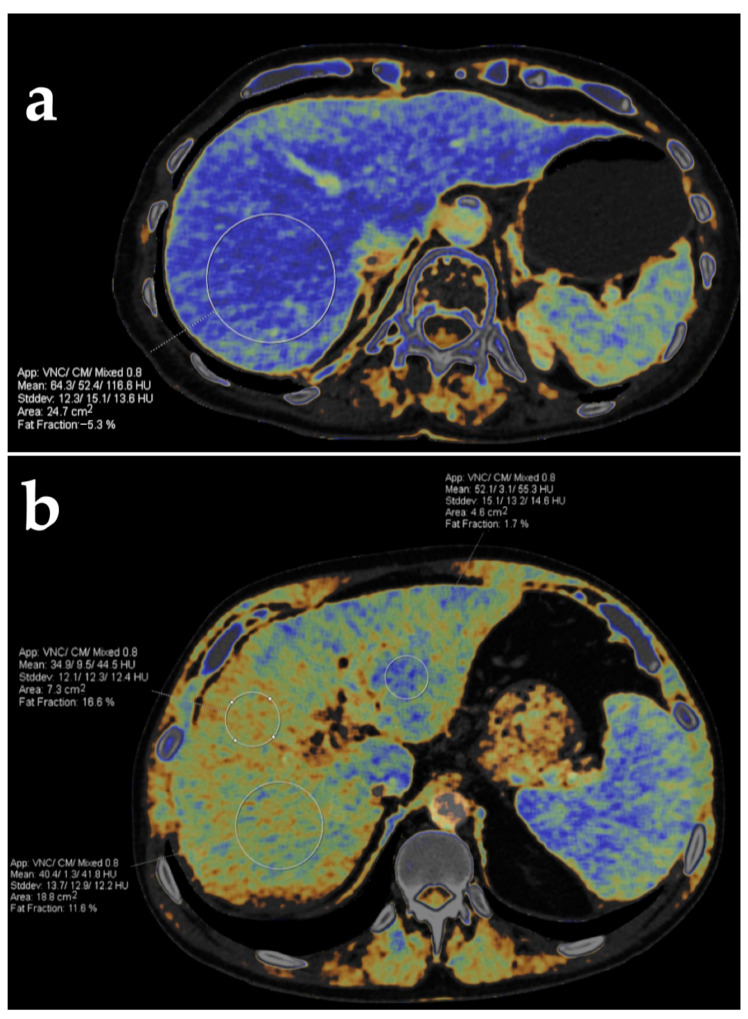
Liver fat quantification using dual-energy CT in two different patients. Fat fraction color-coded maps generated from DECT images in the axial plane show no fat infiltration in the first patient (a) and different fat fractions obtained from three regions of interest in another patient (b).
